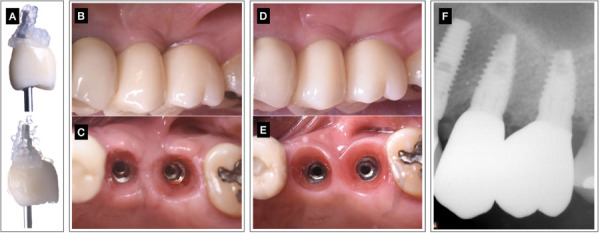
A) Removal of individually cemented/screwed multilayer zirconia crowns (2-5 & 2-6) and blue®m gel appliance before re-inserting them in each of the follow’s controls. B) 6 month follow up of individually cemented/screwed multilayer zirconia crowns rehabilitation at 2-5 and 2-6, buccal view. Observe peri-implant mucosa volume and papillae formation. No black triangle presence. C) Muco-cervical imprint of the crown emergence profile at 2-5, 2-6-zone after 6 months follow up of tissue healing. D) 1 year follow up of individually cemented/screwed multilayer zirconia crowns rehabilitation at 2-5 and 2-6, buccal view. Observe buccal volume improvement. E) Muco-cervical imprint or footprint within ISC of zirconia crowns emergence profile at 2-5, 2-6-zone after 1 year of healing. F) Radiographic control of the definitive implant-crown rehabilitation at 1 year.
